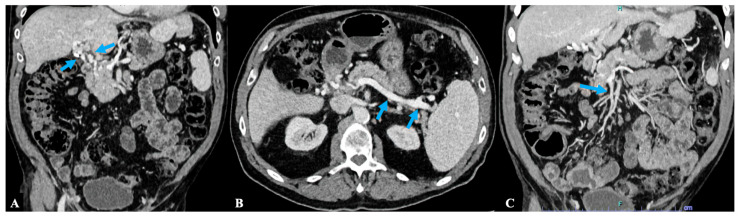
Panels (A–C) show evolution of spleno-mesenteric-portal thrombosis by computed tomography (CT) imaging after 1 year of follow-up, displaying the complete recanalization of previous portal-splenic-mesenteric vein thrombosis with the development of some collateral vein networks. (A) Coronal CT scan in the post-contrast venous phase showing recanalization of the portal circulation through the formation of collateral circles-portal cavernoma (blue arrows). (B) Axial CT scan in the post-contrast venous phase showing complete recanalization of the splenic vein (blue arrows). (C) Coronal CT scan in the post-contrast venous phase showing recanalization of the superior mesenteric vein (blue arrow).
